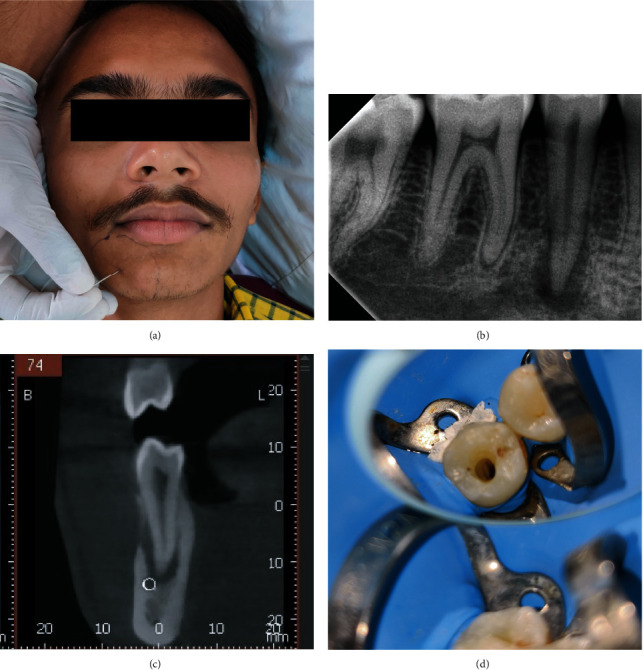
(a) Paraesthesia testing, (b) preoperative RVG, (c) preoperative CBCT, and (d) access cavity under dental dam.
